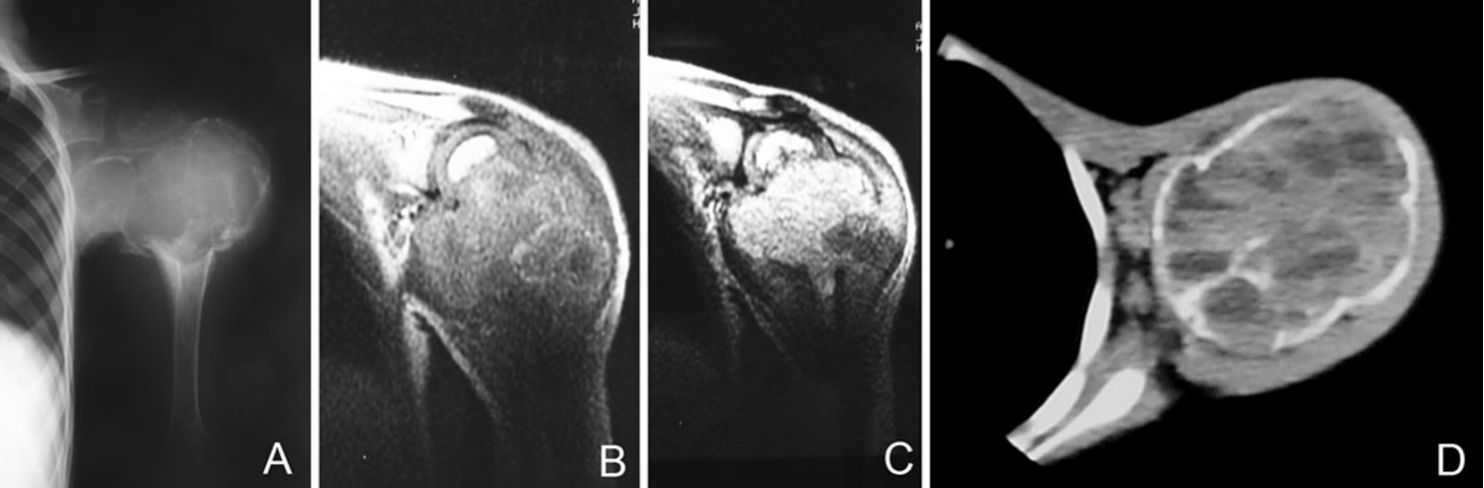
Anteroposterior radiograph (a) and coronal MRI scans (b, c) of the *left shoulder* and *arm* show the blowout appearance of the left proximal humerus. A multiloculated cystic and expansile lesion involving the proximal humeral metaphysis adjacent to the growth plate can be identified. Axial MRI scan (d) indicates a fluid–fluid levels in the cystic lesion
